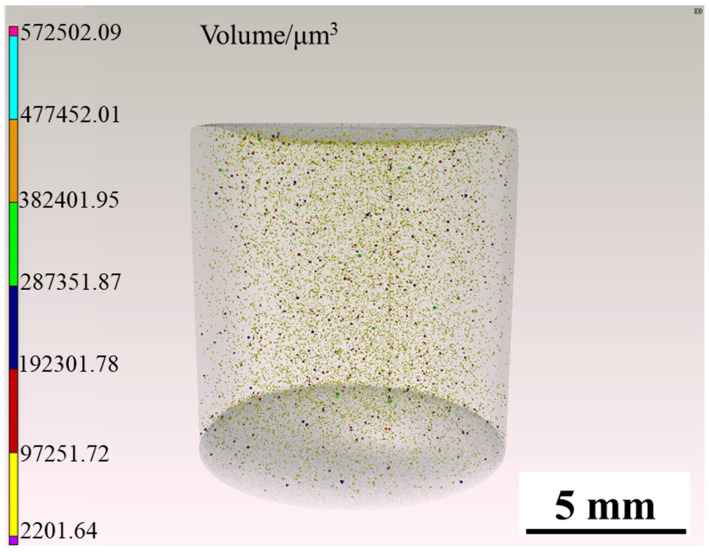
Three-dimensional reconstruction of the A3 sample tested by CT.
